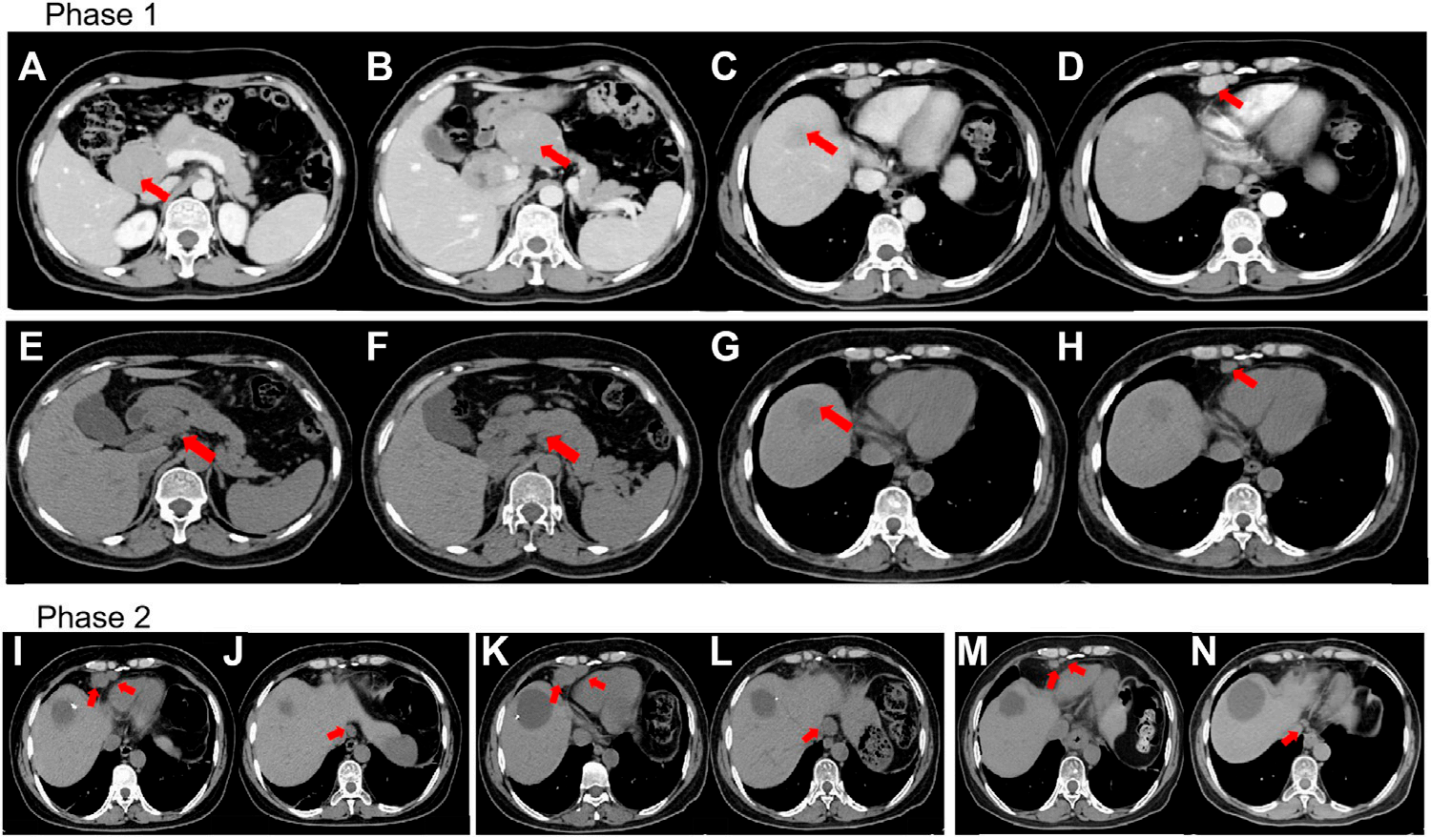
(A–D) Prior to treatment, computed tomography (CT) revealed lesions around the pancreas, liver, and lymph nodes located in the cardiophrenic angle. (E–H) CT revealed that lesions around the pancreas and lymph nodes reduced in size whereas the lesions proximal to the liver remained constant despite four cycles of immunotherapy and chemotherapy (IC). (I,J) CT revealed lymph nodes present even after mesohepatectomy and regional lymph node resection. (K,L) CT showed further enlargement of the lymph nodes in the cardiophrenic angle and paraesophageal region despite two cycles of IC. (M,N) CT revealed shrinkage of lymph nodes after stereotactic ablative radiotherapy. (A,B) and (E,F) The red arrow indicates lesions around the pancreas. (C,G) The red arrow indicates the lesion in the liver. (D,H) The red arrow indicates the lymph nodes in the cardiophrenic angle. (I,K,M) The red arrow indicates the lymph nodes located in the cardiophrenic angle. (J,L,N) The red arrow indicates the paraesophageal lymph nodes.
